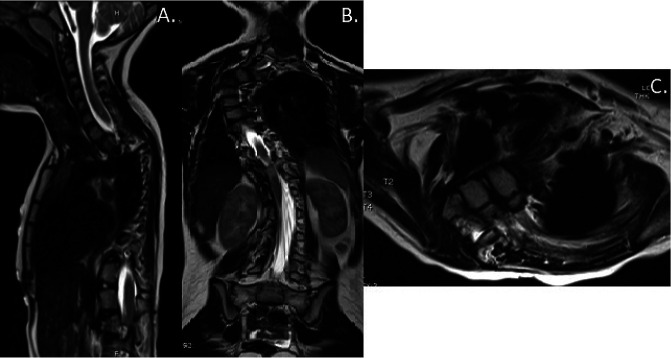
Radiographs showing preoperative magnetic resonance imaging of the patient's early-onset scoliosis. A, Sagittal cut of the cervical, thoracic, and lumbar spine. B, Coronal cut of the cervical, thoracic, and lumbar spine. C, Axial cut of T2, T3, and T4 vertebrae.
